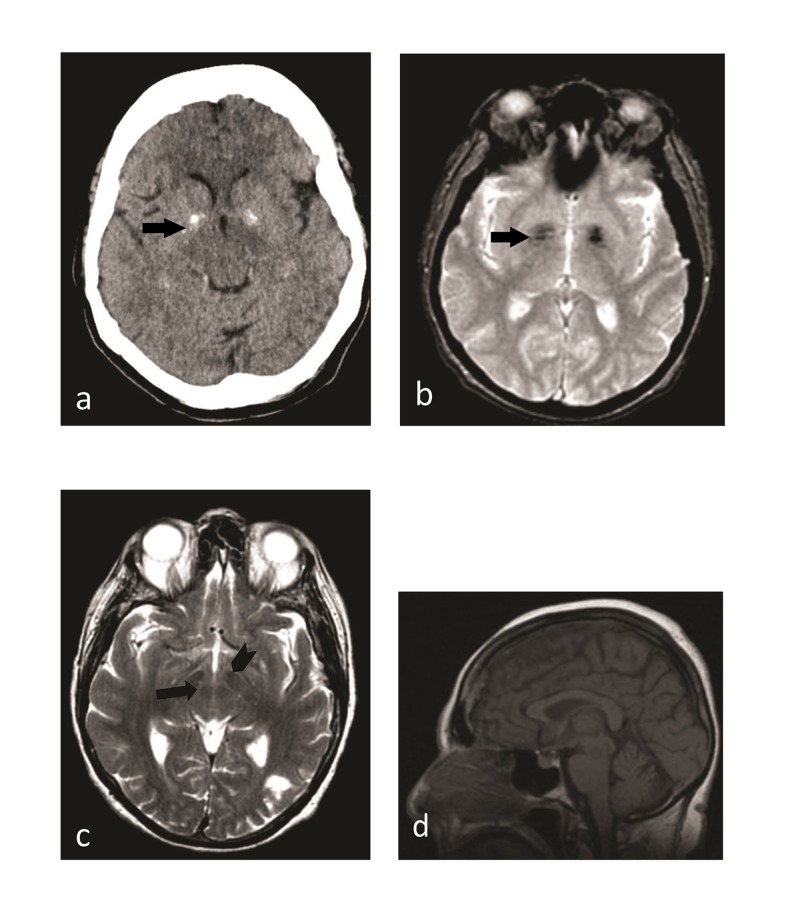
Computed tomography (CT) scan and magnetic resonance imaging (MRI) findings Axial CT image demonstrates (a) mild atrophy and bilateral basal ganglia calcifications (black arrow), which appear hypointense on a corresponding T2W MR image (black arrow); (b) axial T2W image at the level of the midbrain; (c) iron accumulation related hypointensities of the red nucleus (black arrow) and the substantia nigra (black arrowhead). Midline sagittal T1W image shows (d) subtle cortical atrophy, with more pronounced atrophy of the superior vermis.
